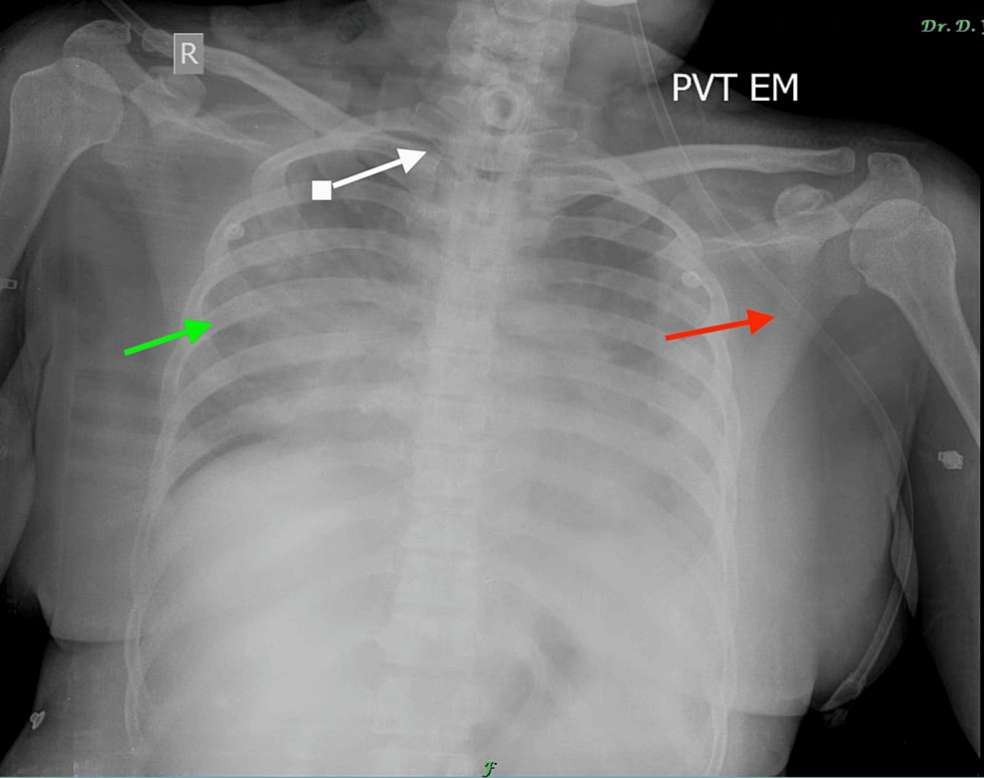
Chest radiograph (anteroposterior view) showing tracheostomy tube in situ (white arrow), nasogastric tube (red arrow) in situ, with right upper lobe consolidation (green arrow) and bilateral cardiophrenic angle blunting. PVT EM: Private Emergency Medicine
